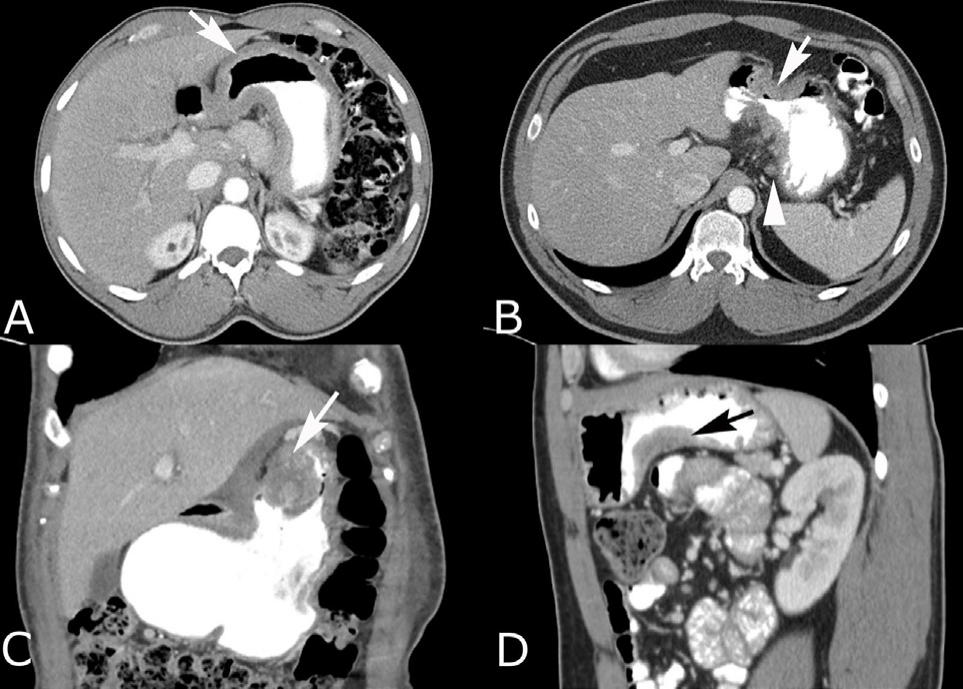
Intravenous and oral contrast-enhanced computed tomography (CT) in four different patients with gastric adenocarcinoma. (A) Diffuse gastric wall thickening with loss of normal rugal fold pattern (arrow). (B) Focal, circumferential narrowing of the antrum with marked wall thickening that has irregular spiculation into the perigastric fat (arrow). Small perigastric lymph node is present (arrowhead). (C) Coronal reconstruction shows an intraluminal polypoid carcinoma (arrow) with heterogeneous enhancement and a soft tissue component that infiltrates the lesser omentum. (D) Sagittal reconstruction shows a carcinoma that is producing focal wall thickening (arrow) along the inferior body of the stomach.
